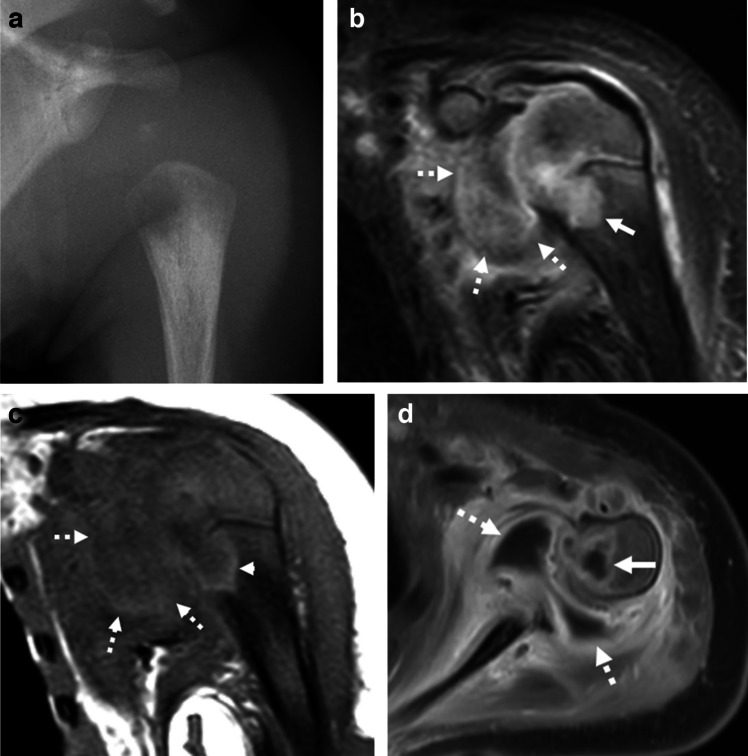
Osteomyelitis and septic arthritis. A 1-month-old boy presenting with decreased use of the left arm. a Frontal radiograph of the left shoulder shows a lytic lesion in the proximal left humeral metaphysis. Coronal T2W FS (b) and T1W (c) MR images show a complex collection within the proximal humeral metaphysis (*solid arrow*), which involves the physis. There is a large glenohumeral joint effusion (*dashed arrows*). Notice the peripheral increased signal on the T1 image (*arrowhead* in part c) consistent with the penumbra sign. d Axial T1W FS post-contrast image shows rim enhancement of the proximal humeral collection, consistent with intraosseous abscess (*solid arrow*), and thick peripheral enhancement of the shoulder joint (*dashed arrows*) related to the septic arthritis
